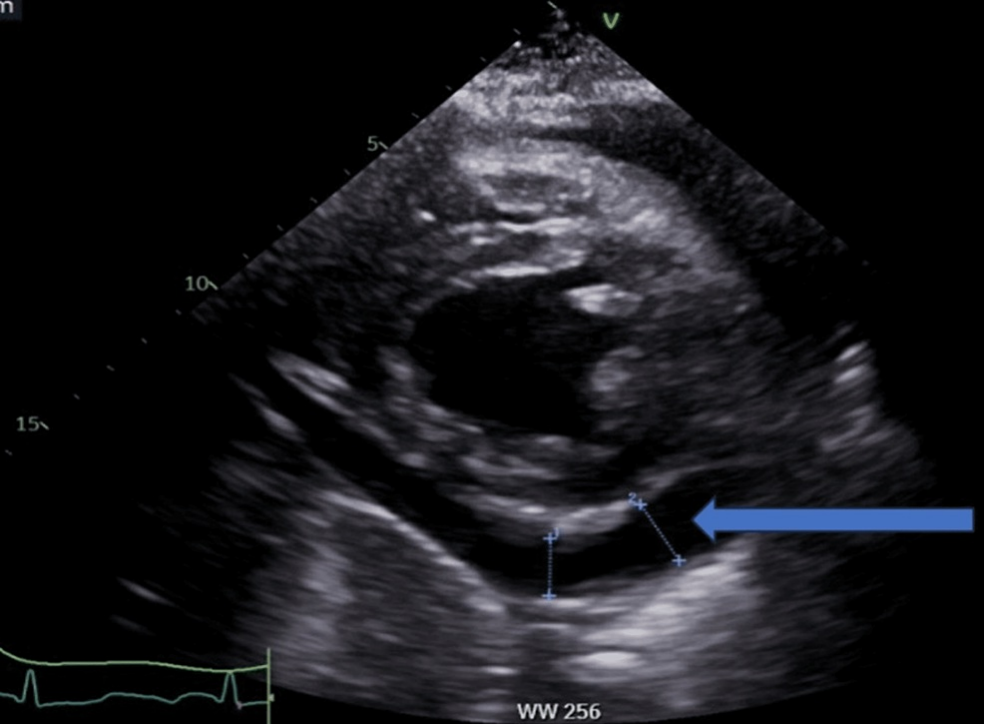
Echocardiogram demonstrating pericardial effusion (blue arrow).
